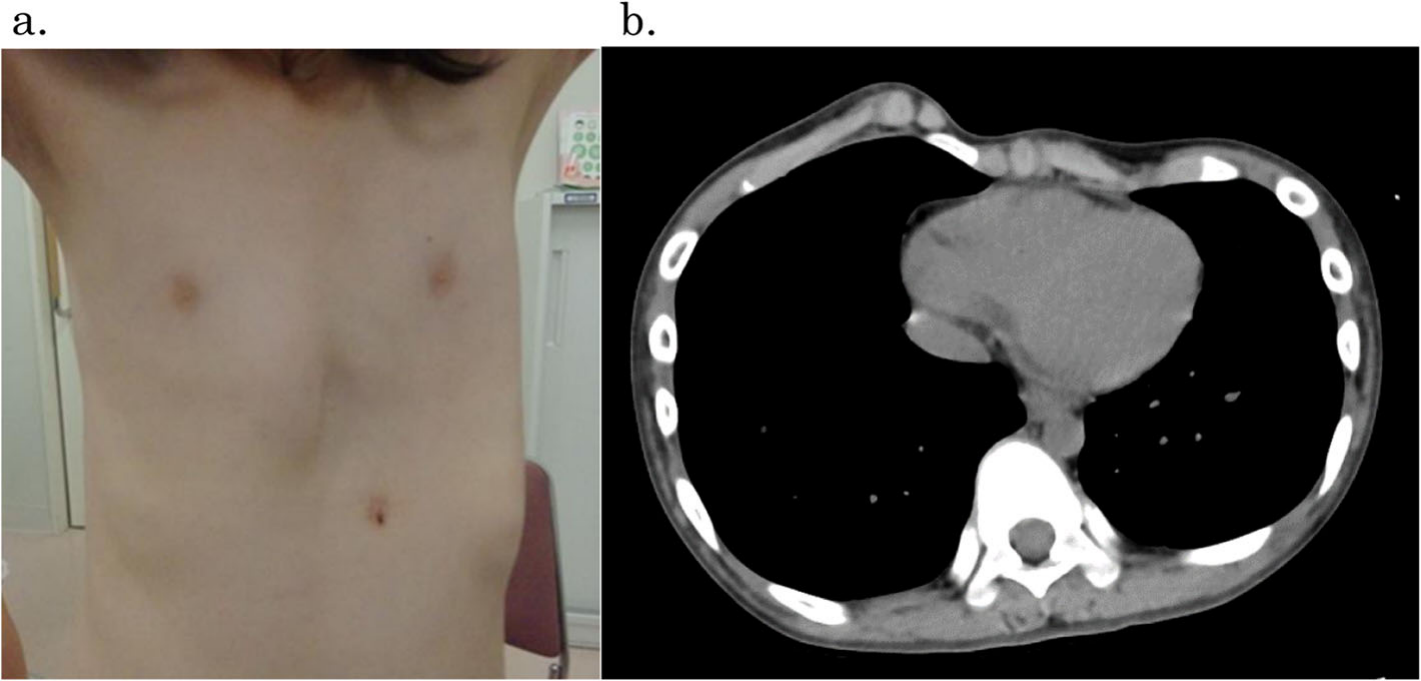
a Appearance. Asymmetric pectus excavatum can be observed. b Preoperative computed tomographic scan of the chest. Computed tomographic scan shows pectus excavatum deformity
